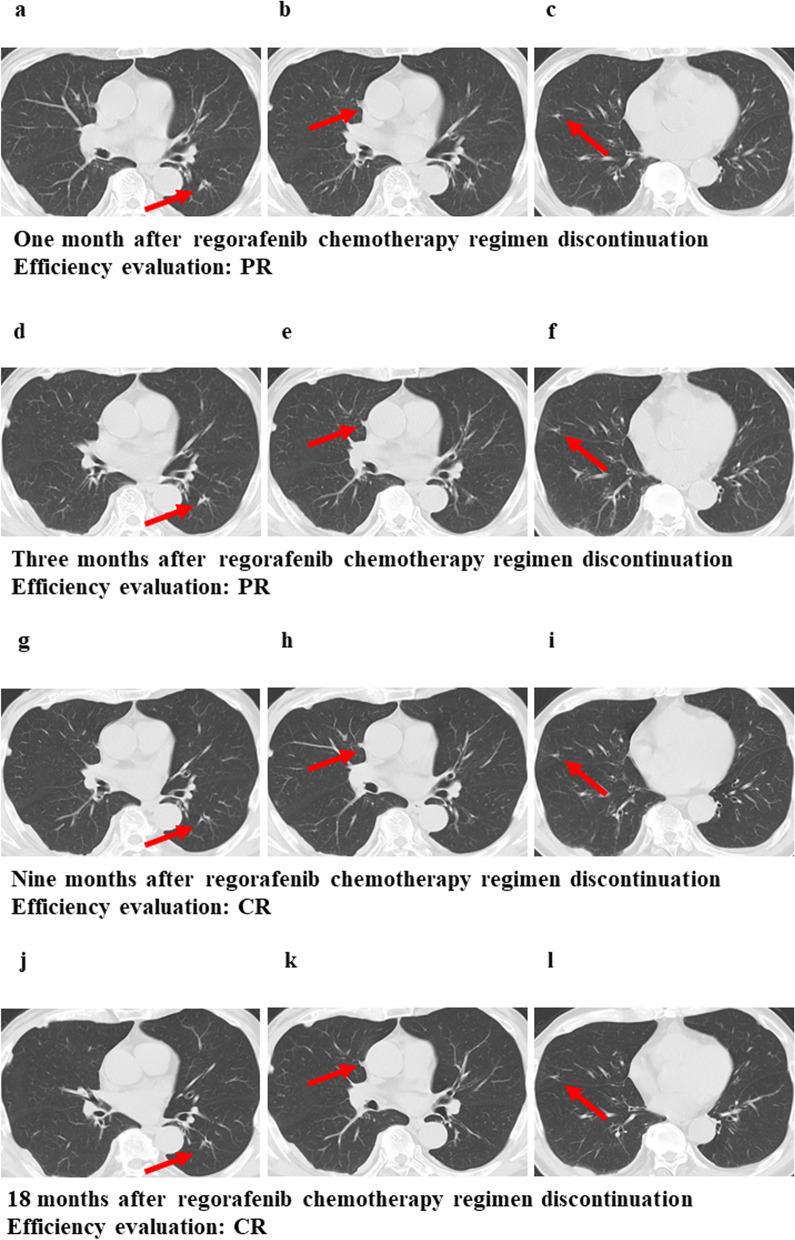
Consecutive CT findings after chemotherapy discontinuation. a–c The lung tumors had shrunk 1 month after chemotherapy discontinuation. d–f The lung tumors had shrunk 3 months after chemotherapy discontinuation. g–i The lung tumors had scarred 9 months after chemotherapy discontinuation. j–l The lung tumors had scarred 18 months after chemotherapy discontinuation. These arrows indicate lung metastases
